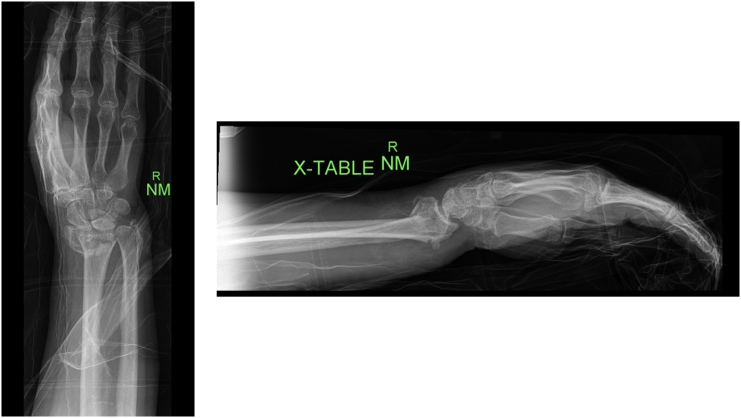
Anteroposterior and lateral injury X-rays demonstrating a comminuted intraarticular distal radius fracture with apex volar angulation, loss of radial height, and loss of radial inclination as well as an associated displaced ulnar styloid fracture.
